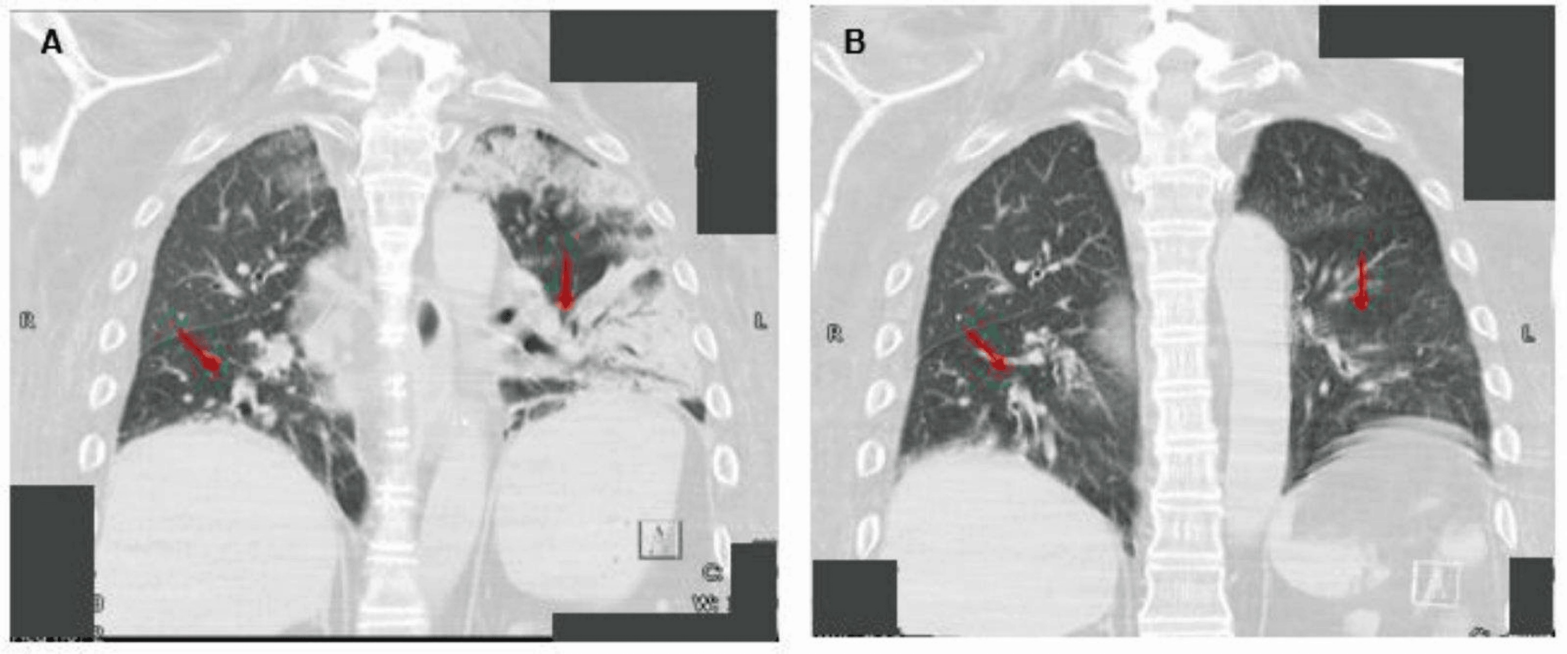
Coronal chest CT images on day 0 and day 21. (A) Coronal CT image on day 0, showing extensive bilateral pulmonary infiltrates, especially in the posterior lower lung fields (arrows).
